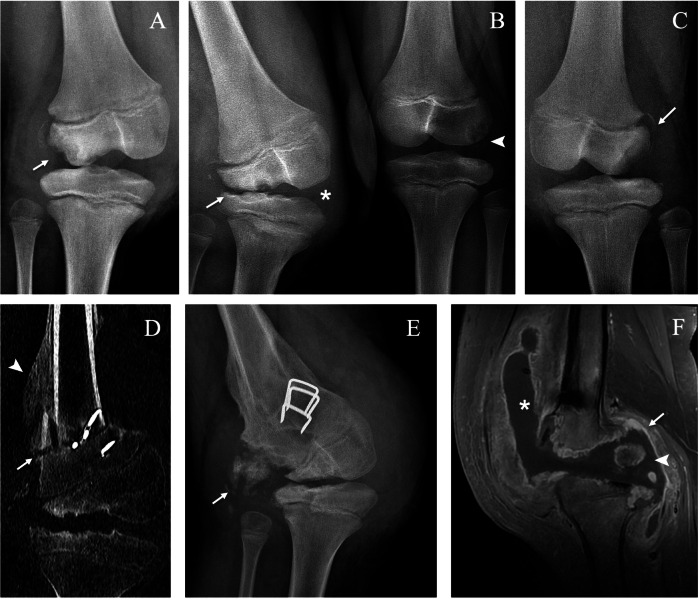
Bilateral knee avascular necrosis (AVN) in a boy with hereditary sensory and autonomic neuropathy type IV. Anteroposterior (AP) radiograph of the right knee at 9 years of age (A) shows bone resorption of the lateral femoral condyle (arrow). AP radiograph of both knees 3 months later (B) shows progression of the right lateral condyle resorption (arrow) with valgus deformation and widening of the medial articular space (asterisk) and lytic changes of the left lateral condyle (arrowhead). AP radiograph of the left knee after 3 more months (C) demonstrates progression of the osteolysis (arrow). Coronal CT of the right knee (D) after distal femoral varus osteotomy (arrow) shows signs of consolidation (arrowhead). AP radiograph of the right knee 1 year after surgery (E) shows recurrence of AVN of the lateral condyle (arrow). Sagittal T1 post-gadolinium MRI (F) during an episode of septic arthritis shows nonspecific findings such as joint effusion (asterisk), synovial enhancement (arrow) and intra-articular loose bodies (arrowhead)
